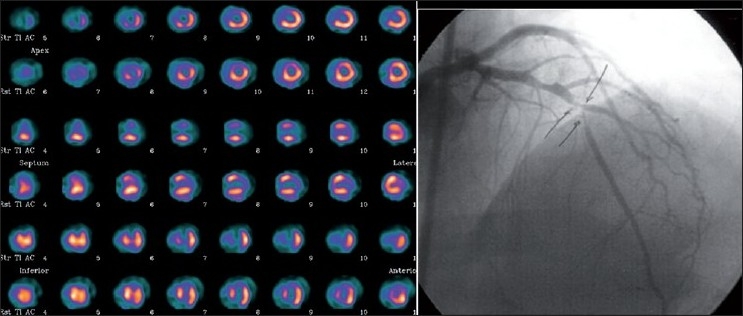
Myocardial perfusion scintigraphy in a patient with 90% LAD stenosis. Reversible ischemia involving > 10% of the LAD territory is noted. The patient was subsequently revascularized considering the significant viable, but ischemic myocardium in the LAD territory
